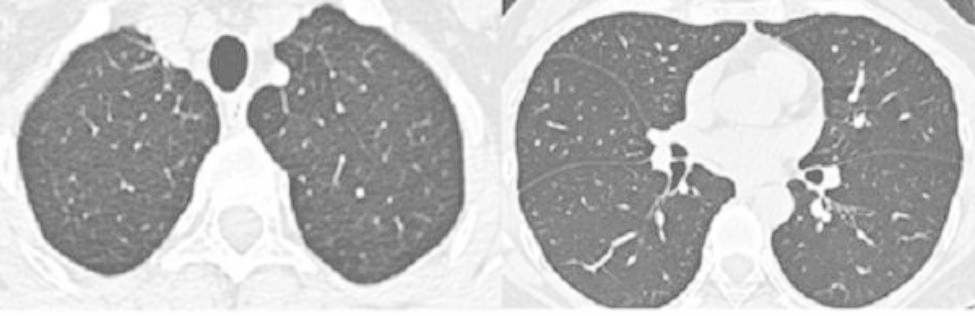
After 1 month of anti-tuberculosis treatment, the patient ‘s shortness of breath was completely relieved, chest CT was reviewed, and the right pneumothorax was completely resolved
